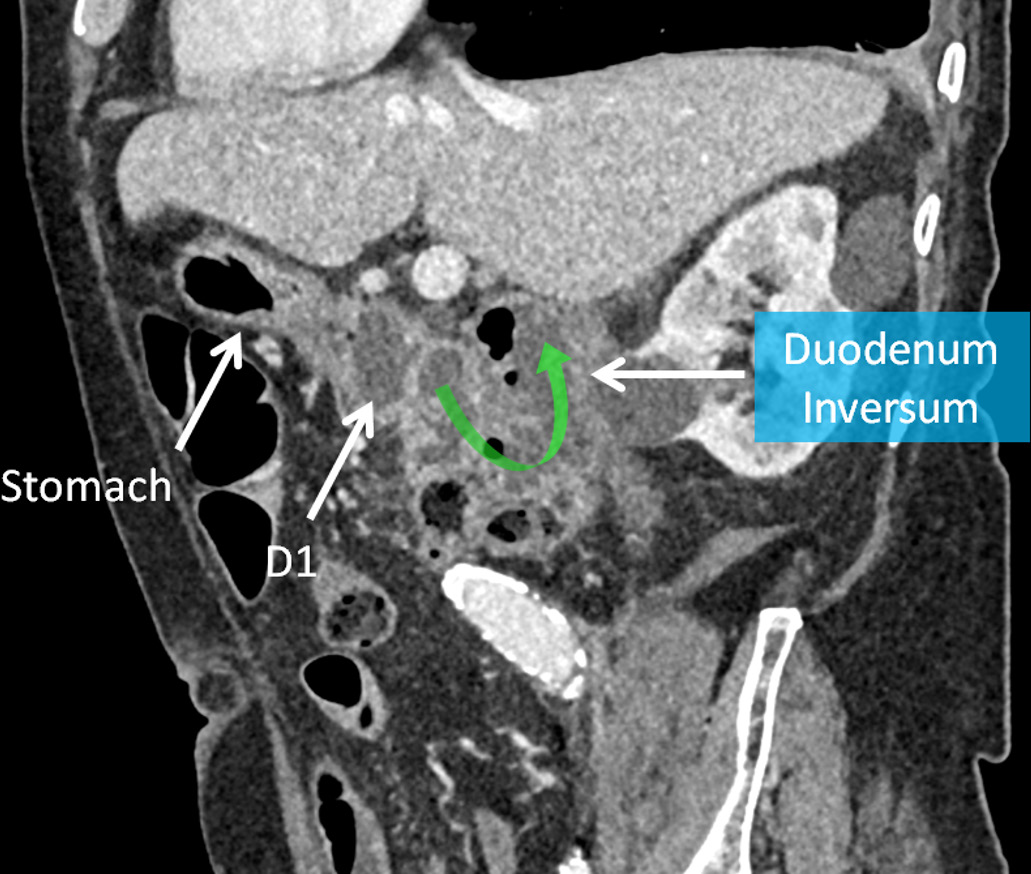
CT multiplanar reconstructed oblique sagittal image demonstrating duodenum inversum with the proximal duodenum travelling posteriorly and superiorly prior to crossing midline.
